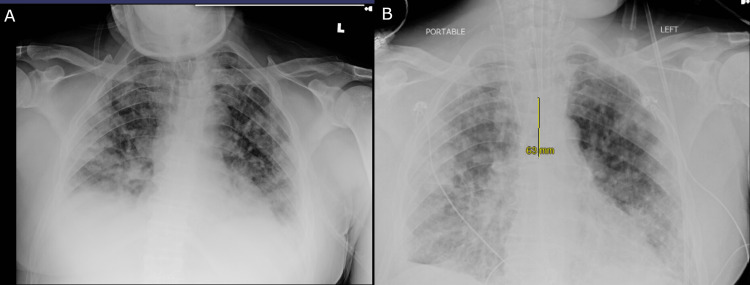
Anterior/posterior chest X-rays AP chest X-rays demonstrated bilateral hazy airspace disease with probable underlying pleural effusions, suggestive of multifocal pneumonia. Films were taken before (A) and after (B) endotracheal intubation. AP: anterior/posterior
